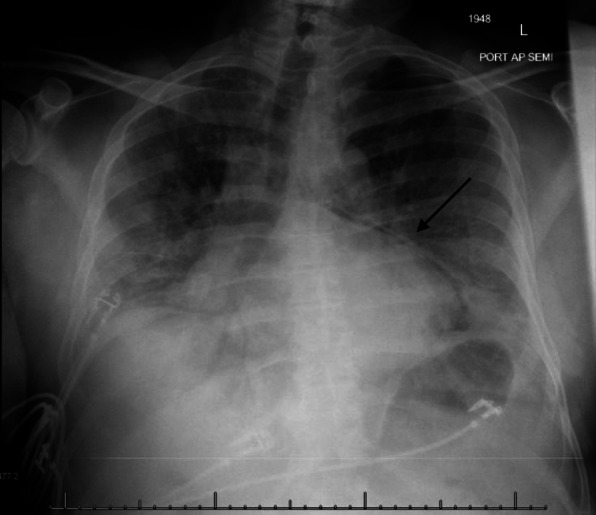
A frontal chest radiograph of Patient 2 showing interval development of spontaneous pneumomediastinum and possible pneumopericardium (arrow)
